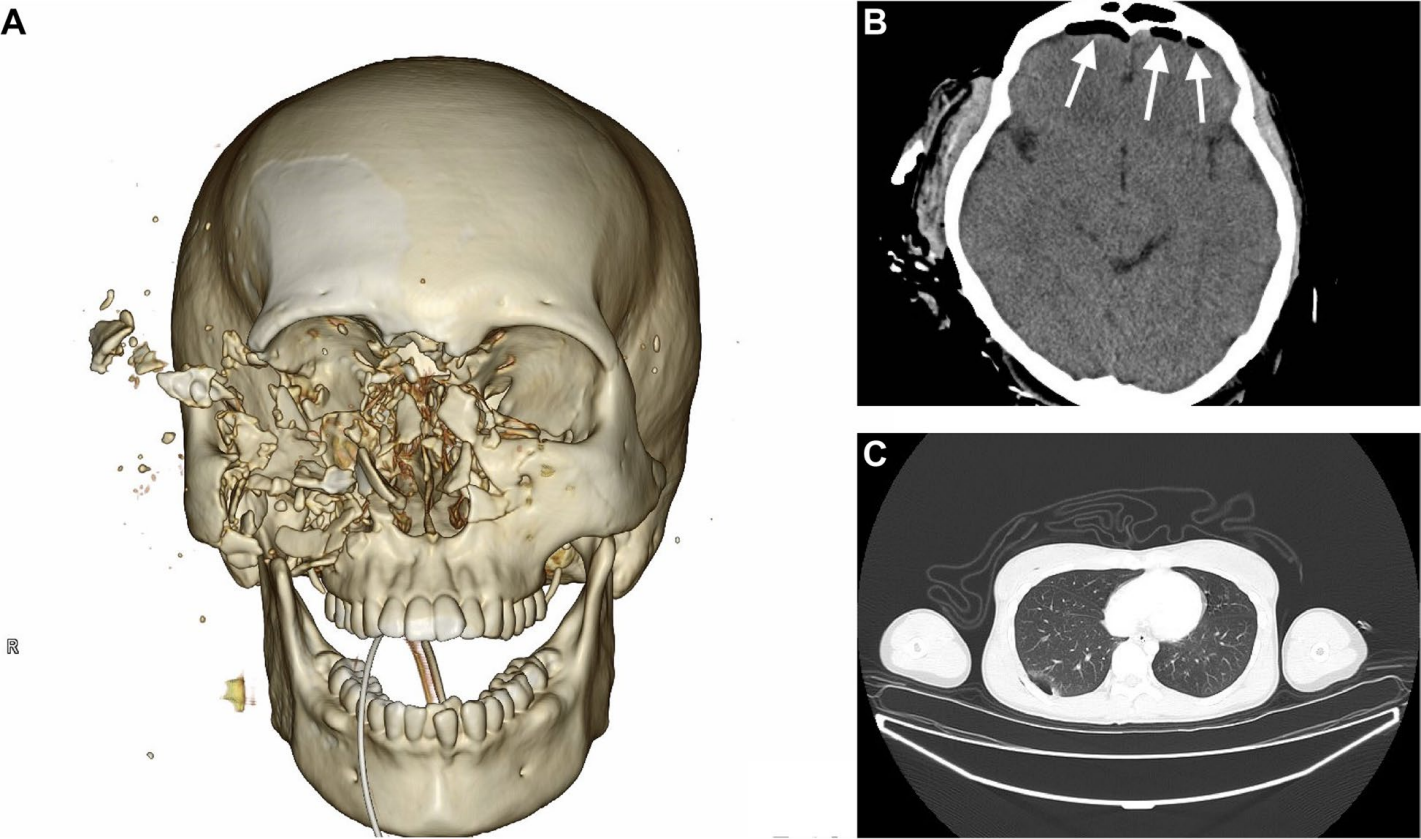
Computed tomography (CT) images. A 3D reconstruction of the initial CT scan showing multiple open facial fractures. B Head CT scan showing pneumoencephalopathy due to disruption of the frontal sinus (arrow). C Chest CT scan showing a small pneumothorax on the right side
